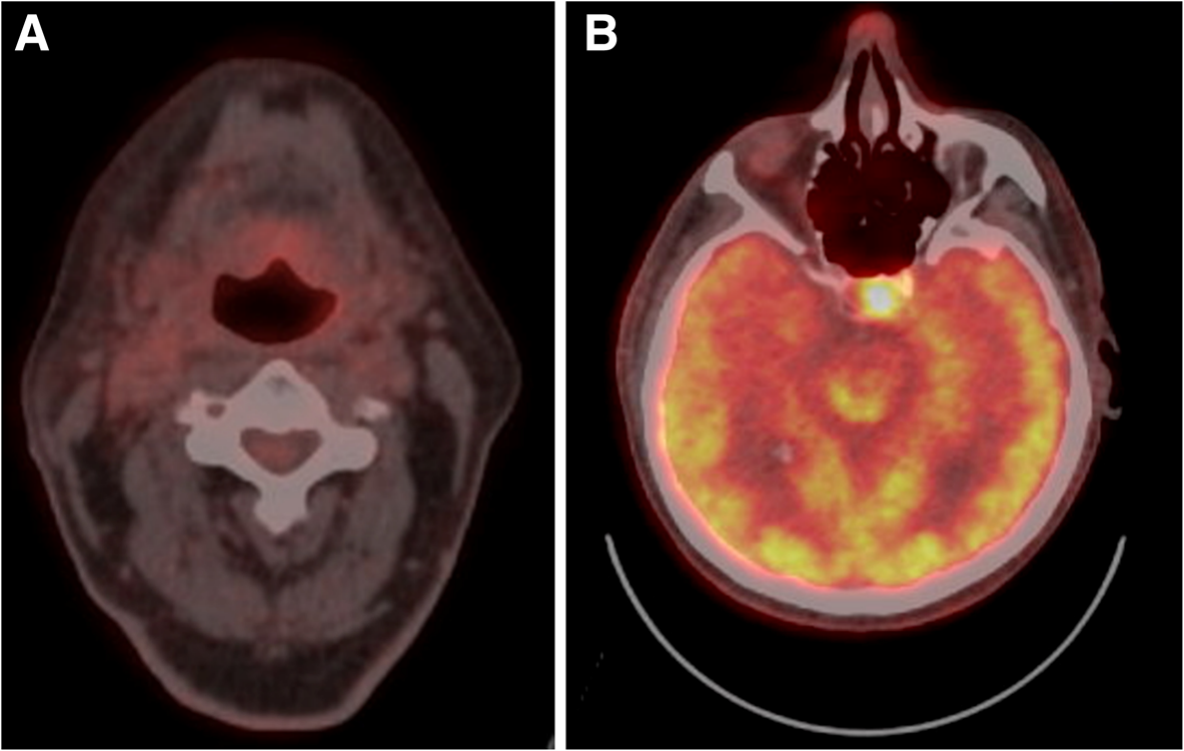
PET scan demonstrating resolution primary tumor and new sella lesion. PET demonstrating a metabolic resolution of the primary tumor and b intense focal FDG uptake within the left sella
